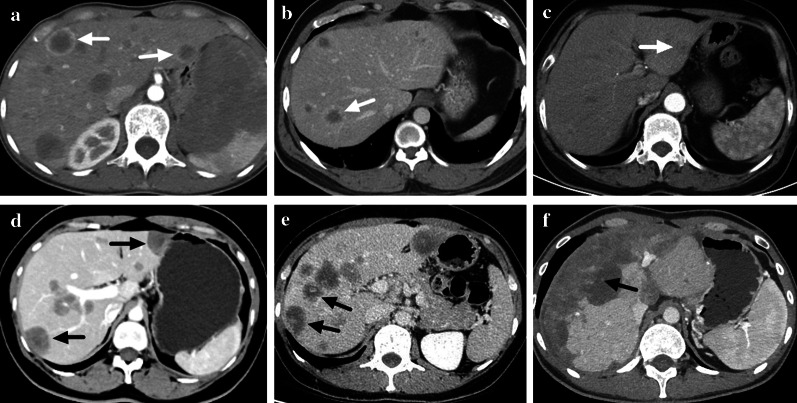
Enhancement patterns of HEH lesions on contrast-enhanced CT scan. a Ring-like enhancement on arterial phase (marked with white arrows). b Ring-like enhancement on portal phase (marked with a white arrow). c Target-like enhancement on arterial phase (marked with a white arrow). d Target-like enhancement on portal phase (marked with black arrows). e Core enhancement on portal phase (marked with black arrows). f Heterogenous enhancement on portal phase (marked with a black arrow)
